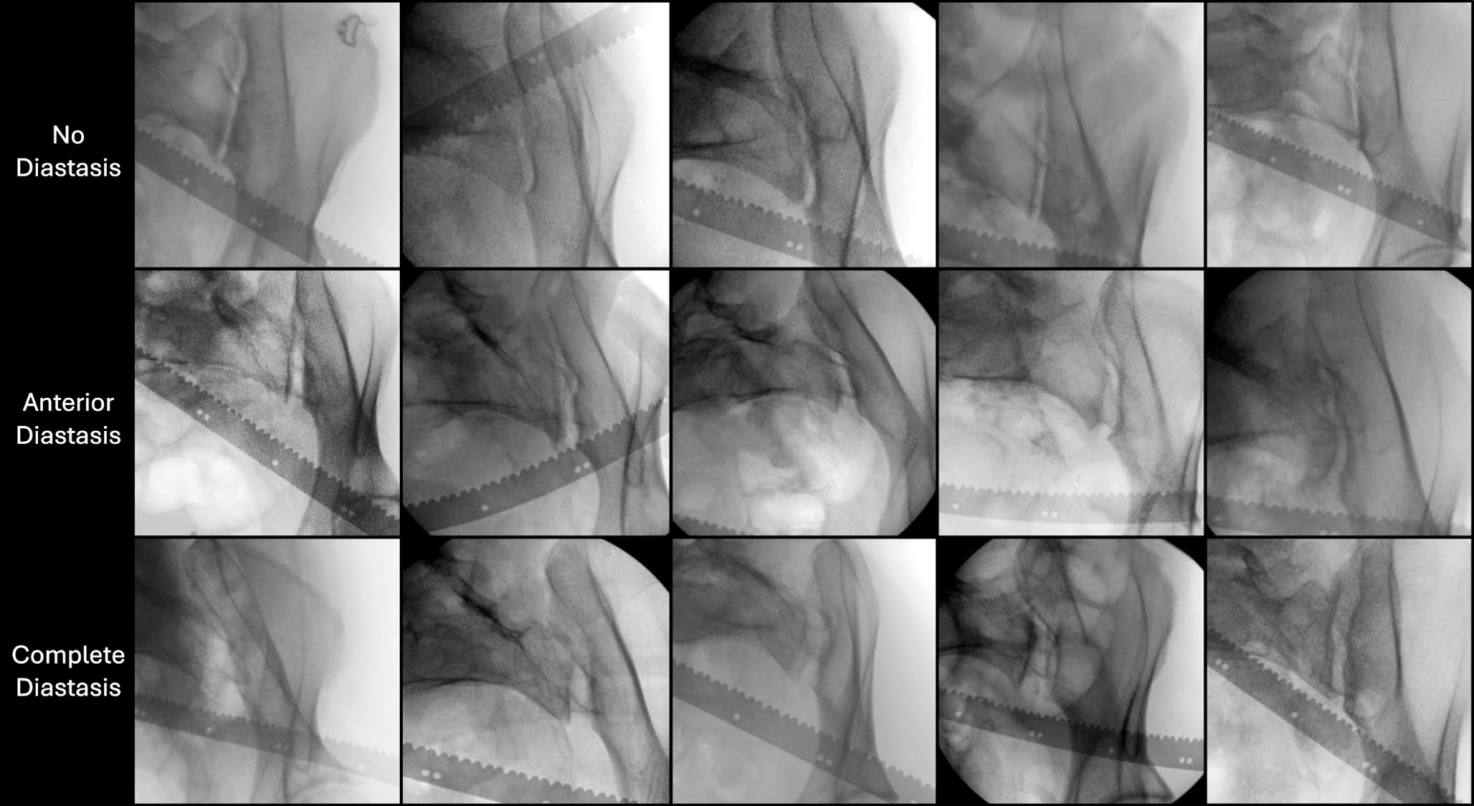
Examples of radiographs of the sacroiliac (SI) joints taken in the external rotation stress maneuver from the obturator inlet projection, with either no diastasis (row 1), anterior diastasis (row 2) or complete diastasis (row 3). As seen in the figure, patients with no SI diastasis had little to no displacement of the ilium, patients with anterior SI diastasis had an ilium that pivoted laterally around the posterior SI joint, indicating intact posterior ligaments, and patients with complete SI diastasis presented with a laterally displaced ilium
